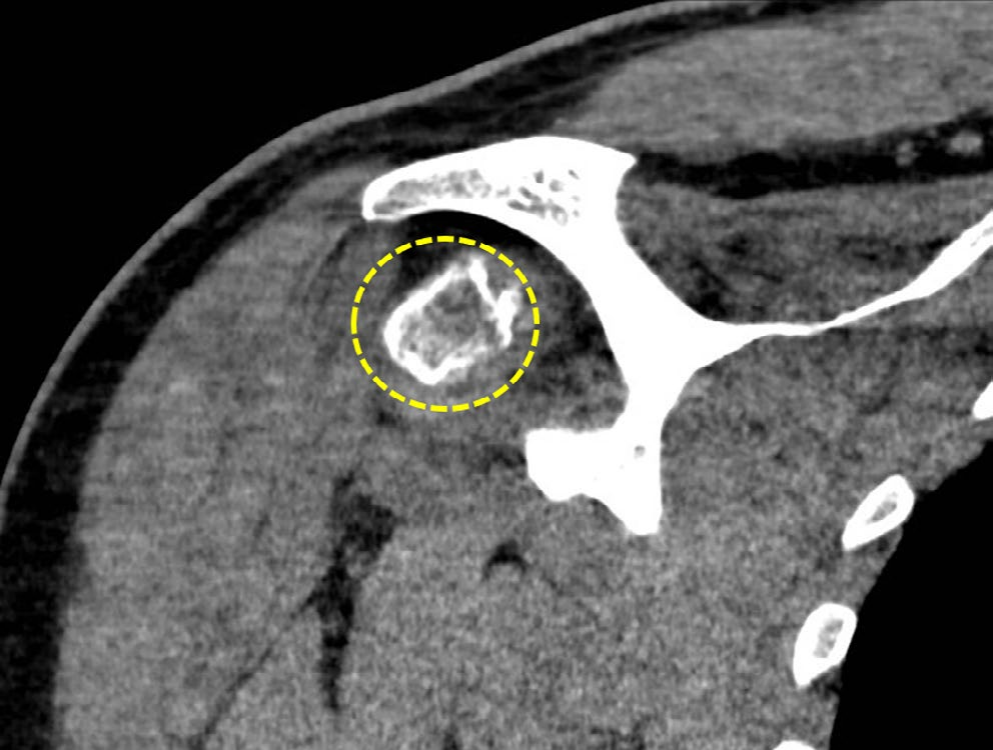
Computed tomography image (soft tissue window) showing the extension of the mass at the coronal reformation (yellow circle). Peripheral mineralization of the lesion is demonstrated. No soft tissue edema is observed
